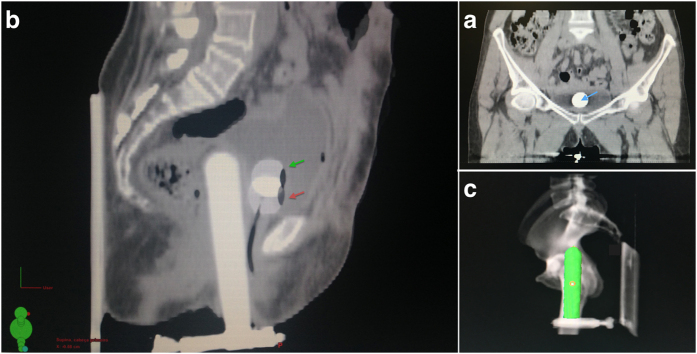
(a) Sagittal view of the fused planning CT scan at both tension levels: standard (position A, green arrow) and extra tension (position B, red arrow). (b) Bladder reference point identified at the center of the balloon on a coronal plane (blue arrow). (c) Digitally reconstructed radiograph (DRR) from the CT scan in position A.
